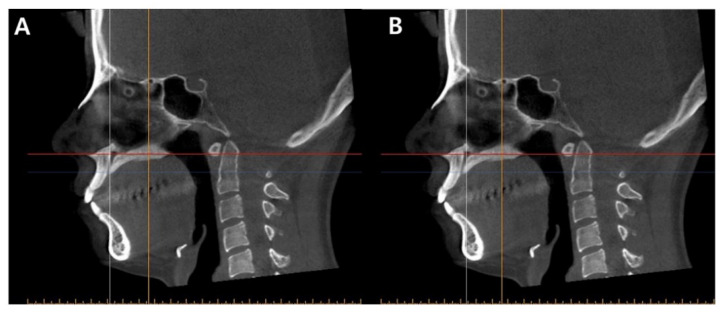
Sagittal view for bone density measurements: (A) Cone-beam computed tomography (CBCT) image was oriented so that the white vertical line was placed along the posterior border of the incisive foramen at an exit of the inferior canal. (B) The white vertical orientation line was then moved posteriorly by 1.56 mm to create the anterior limit for data collection.
